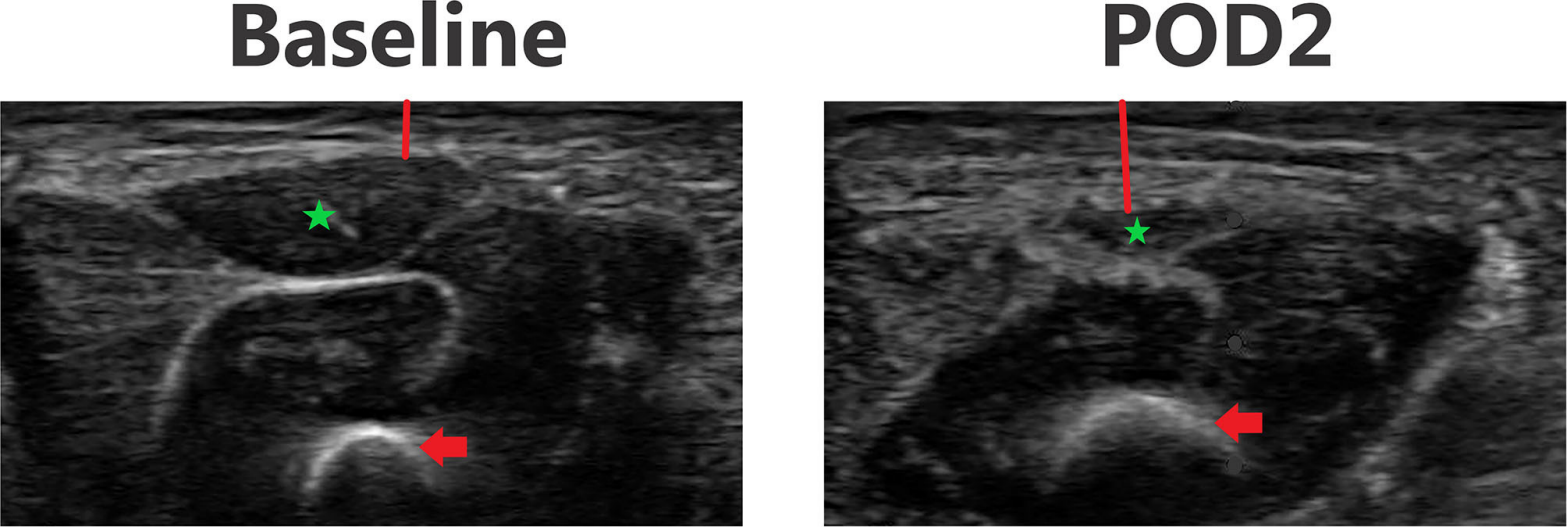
Point-of-care ultrasound images of anterior thigh at baseline and POD 2 of the same patient. Both images are presented on identical scales (total depth of 1.8 cm from the skin). Red vertical line demonstrates subcutaneous tissue thickness—there is a marked increase in subcutaneous tissue seen on POD 2. Red arrow—femur bone. Green star—echogenic muscle changes seen on POD 2.
